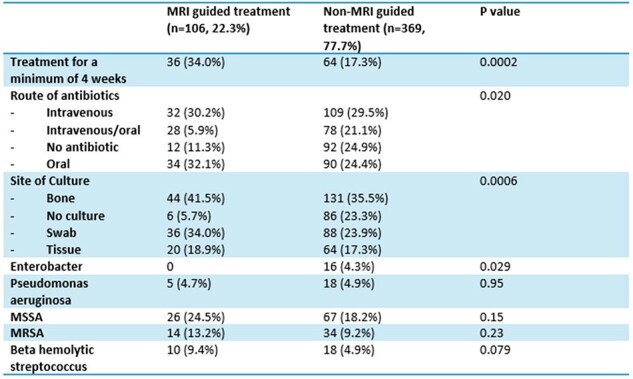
Treatment Characteristics MRI=magnetic resonance imaging, MSSA=methicillin susceptible Staphylococcus aureus, MRSA=methicillin resistant Staphylococcus aureus
